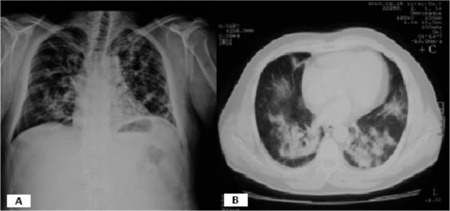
Chest X-ray showed bilateral interstitial infiltrates (A). Thoracic CT revealed patchy parenchymal consolidations in both apical lungs, bilateral superior segments of the lower lobes, and the posterior regions of both lungs (B).
